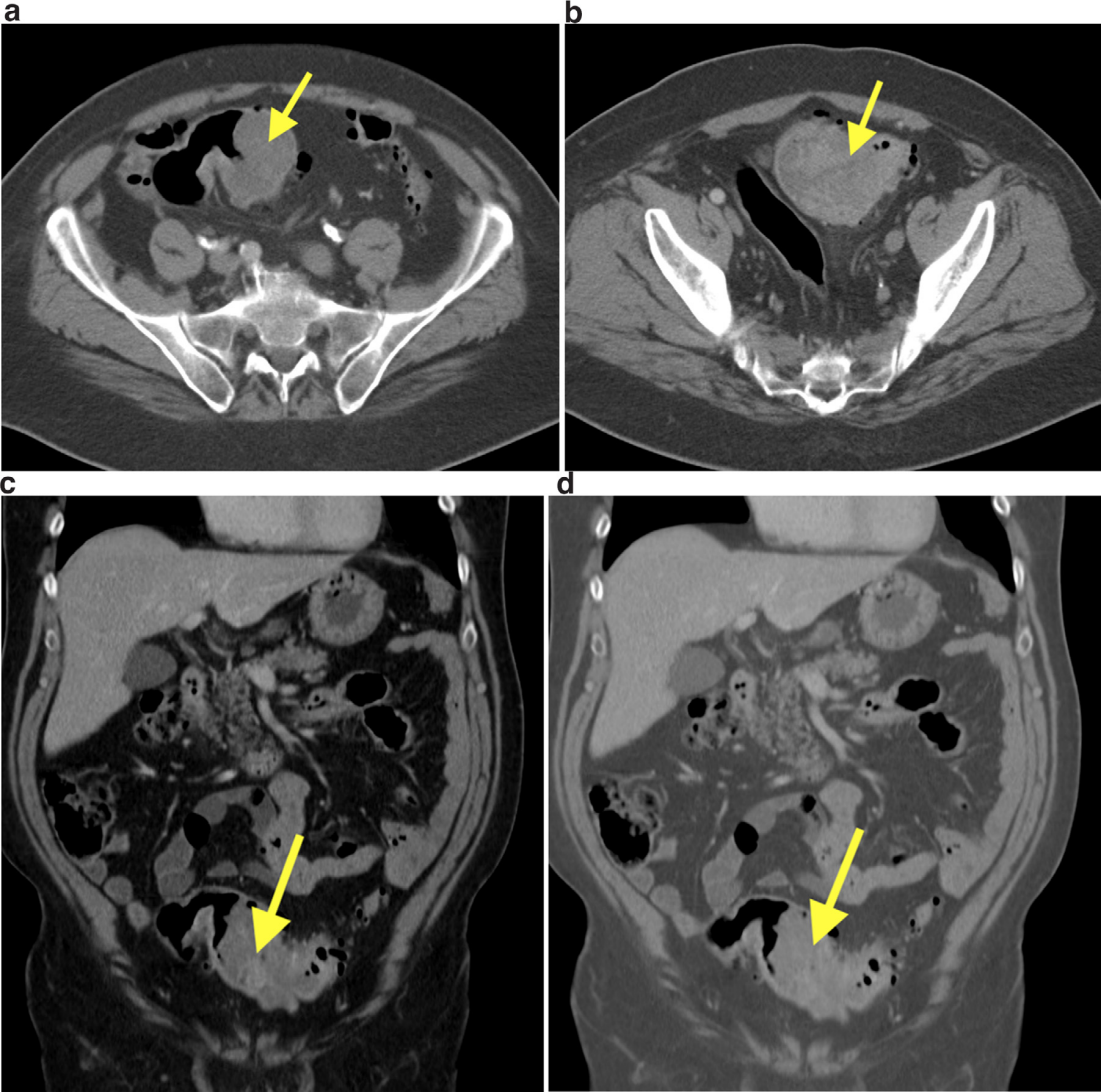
(A,B) CT scan done with IV contrast material demonstrates a 10 cm mass (arrows) in the sigmoid colon with areas of tumor necrosis but no perforation. (C,D) CT scan in coronal plane and 3D volume rendering demonstrates the lobular mass (arrows) that has areas of necrosis. The tumor is not obstructing the bowel.
